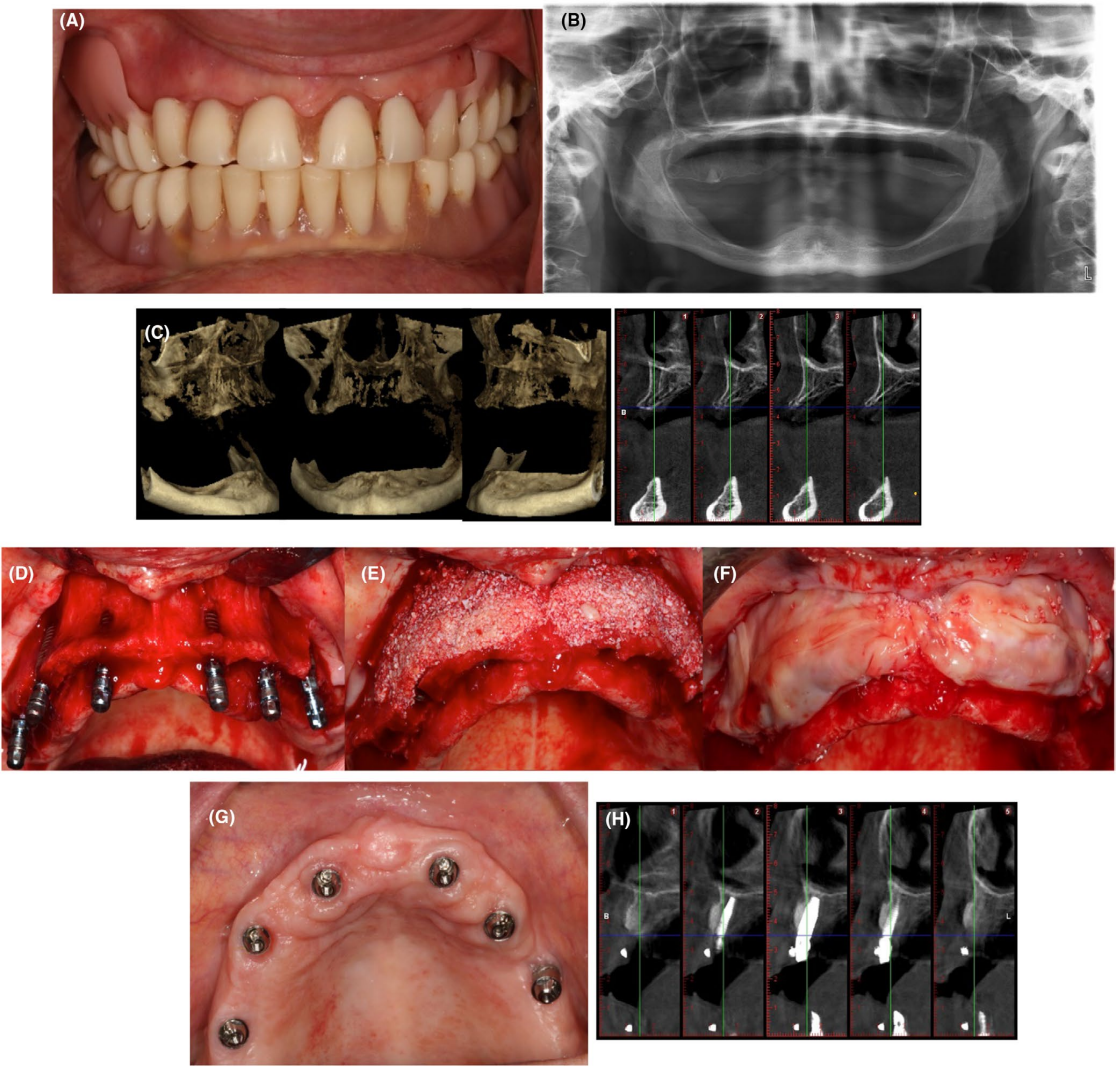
Clinical case of simultaneous horizontal bone augmentation and implant placement. (A) 55‐year‐old female patient had a fully edentulous upper arch with poor bone quantity and quality (Class 3). (B) Preoperatory panoramic image. (C) Preoperatory cone‐beam computed tomography (CBCT). (D) Surgical approach for implant placement. (E and F) Horizontal bone augmentation with leukocyte‐ and platelet‐rich plasma (L‐PRF) bone block (xenograft, chopped pieces of two L‐PRF membranes, and liquid fibrinogen) covered with L‐PRF membranes. (G) 1‐year follow‐up. (H) 1‐year follow‐up CBCT.
